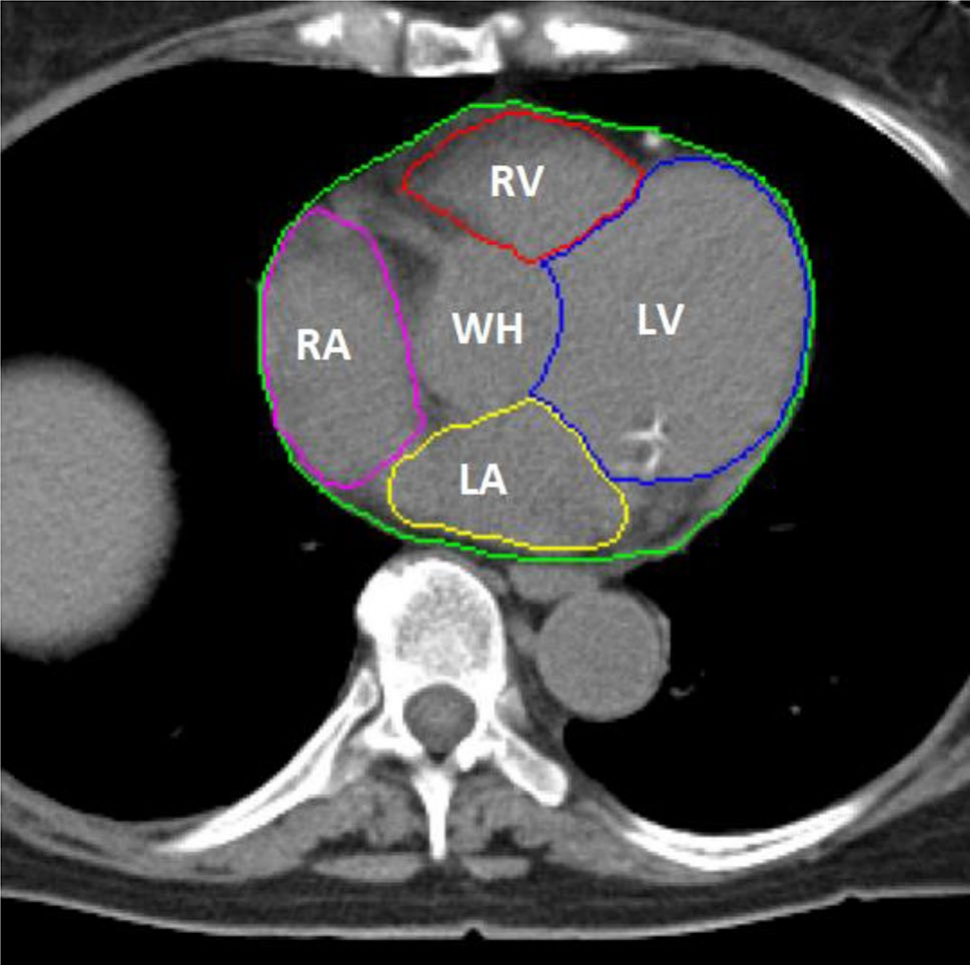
CT image with contours of the investigated structures as made by the experienced cardiac radiologist and physician assistant specialized in breast cancer: whole heart(WH) (green), left ventricle (LV) (blue), right ventricle (RV) (red), left atrium (LA) (yellow), right atrium (RA) (purple)
